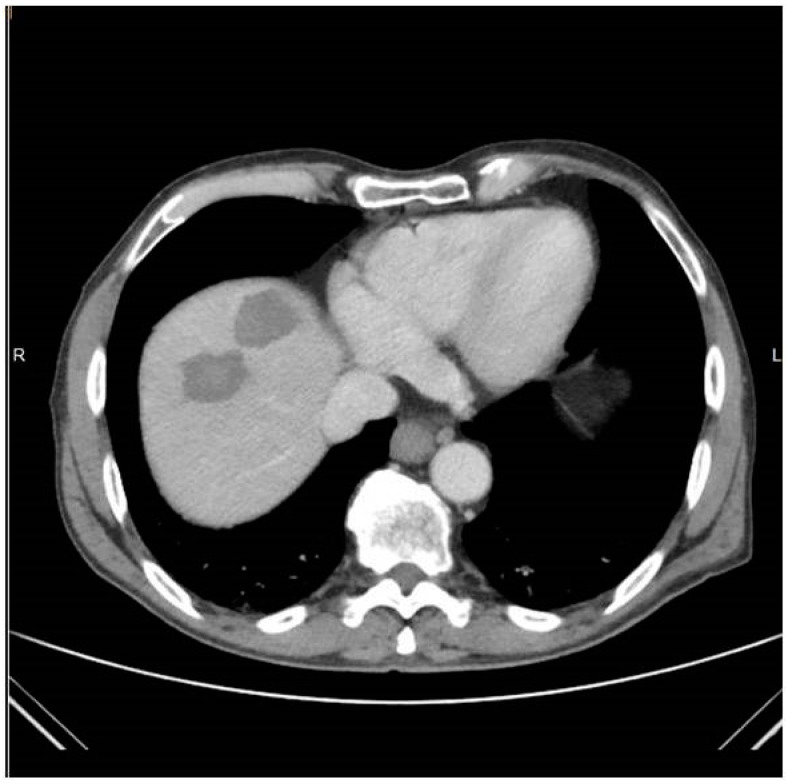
Same patient as in Figure 1: Computed tomography axial scan post intravenous contrast medium injection in the portal venous phase immediately post ablation evaluating the ablation zone and desired safety margins.
